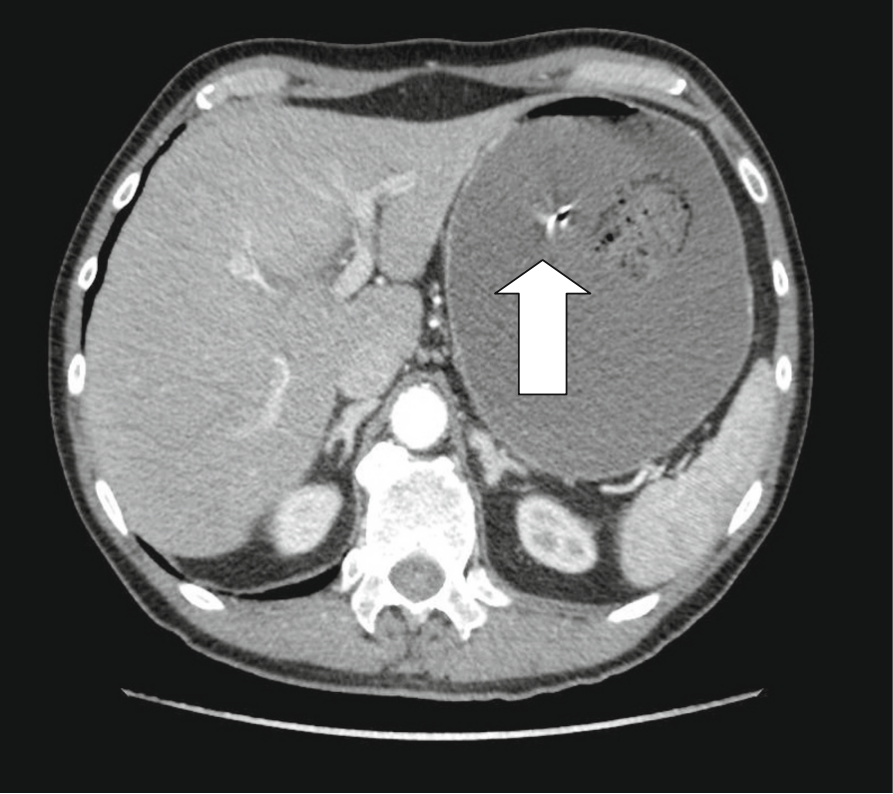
Preoperative abdominal computed tomography scan. The *arrow* indicates the intraoperative view of gastric phytobezoar while removing
